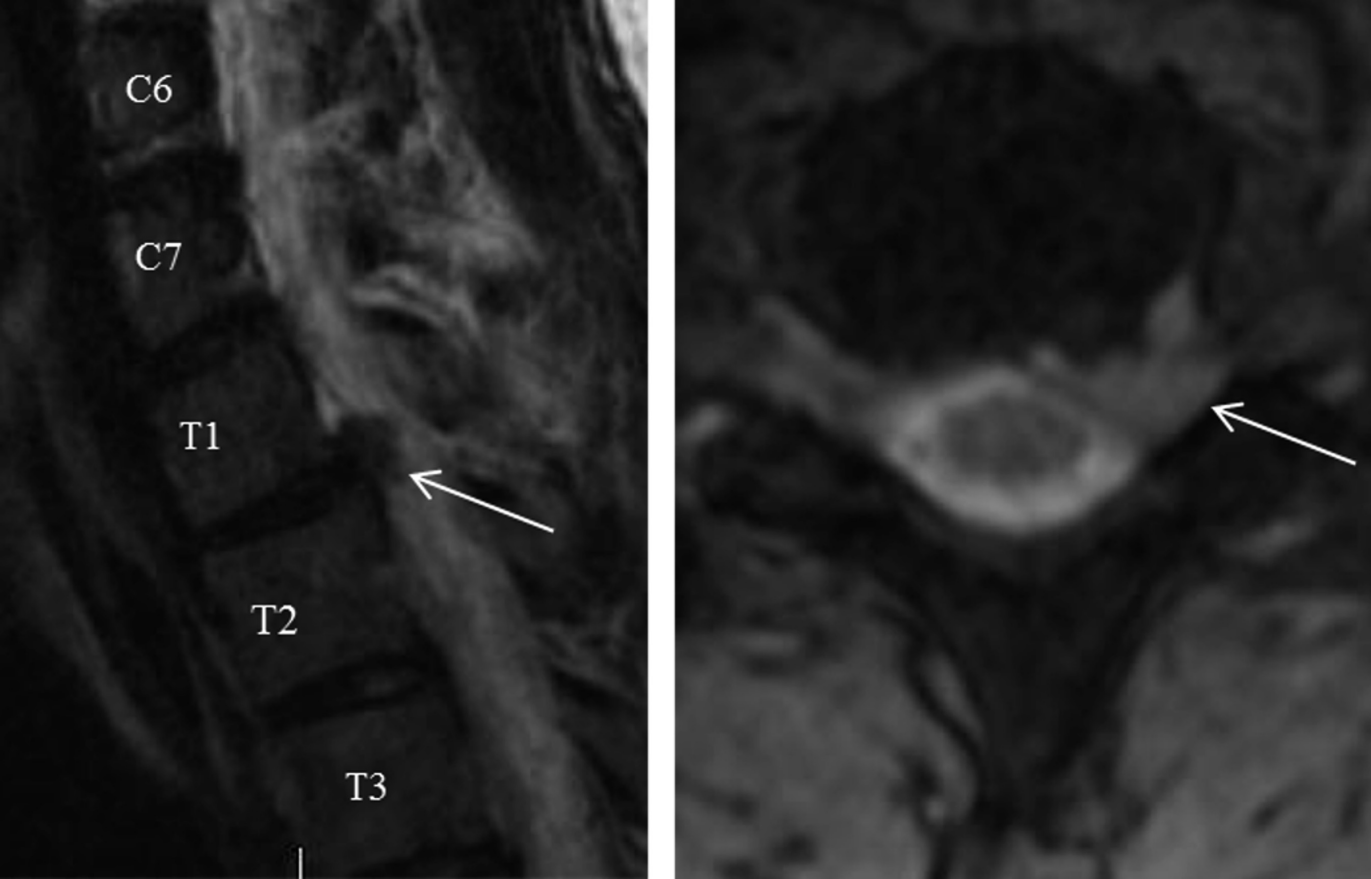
T2 sagittal and axial MR images with T1-T2 disk herniation (arrows).
